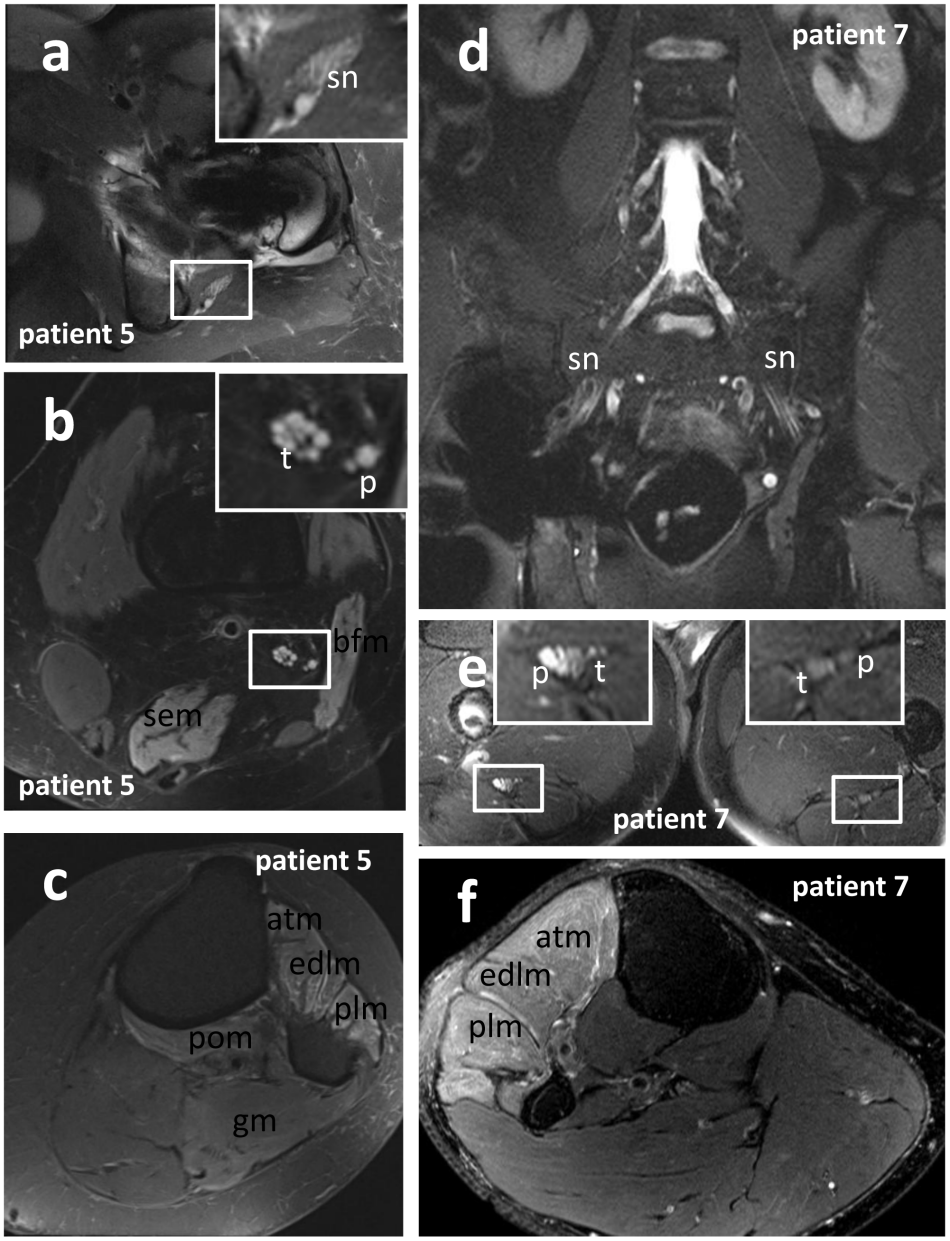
Localization and fascicular distribution of T2-nerve lesion, and denervation pattern of target muscles. siMRN in patient 5 (a, b, c: transversally orientated T2-weighted TSE with fat-suppression) and patient 7 (d: coronally orientated T2 STIR; e, f: transversally orientated T2-weighted TSE with fat-suppression). Depending on the extent of implant-related artifacts, evaluation of the sciatic nerve may be impaired or even impossible for a certain region (d). Regular depiction of the nerve roots and lumbar pexus in patient 7 (d), but the subtrochanteric sciatic nerve exhibits a peroneally accentuated T2-lesion (e), and the peroneally innervated muscles of the proximal lower leg show signs of denervation (f). In patient 5 the proximal sciatic nerve showed a T2-lesion in proximity of the ischial tuberosity (a), affecting all fascicles of the nerve at level of the hip (a) and thigh (b). Furthermore the denervation of the biceps femoris and semimembranosus muscle of the thigh (b), and the anterior tibialis, extensor digitorum, peroneus longus, popliteus and gastrocnemius muscle of the lower leg (c) indicate affection of the tibial and peroneal division of the sciatic nerve. Abbreviations: atm: anterior tibialis muscle; bfm: biceps femoris muscle; edlm: extensor digitorum longus muscle; gm: gastrocnemius muscle; p: peroneal portion of the sciatic nerve or peroneal nerve; plm: peroneus longus muscle; pom: popliteus muscle; sem: semimembranosus muscle; sn: sciatic nerve; t: tibial portion of the sciatic nerve or tibial nerve.
